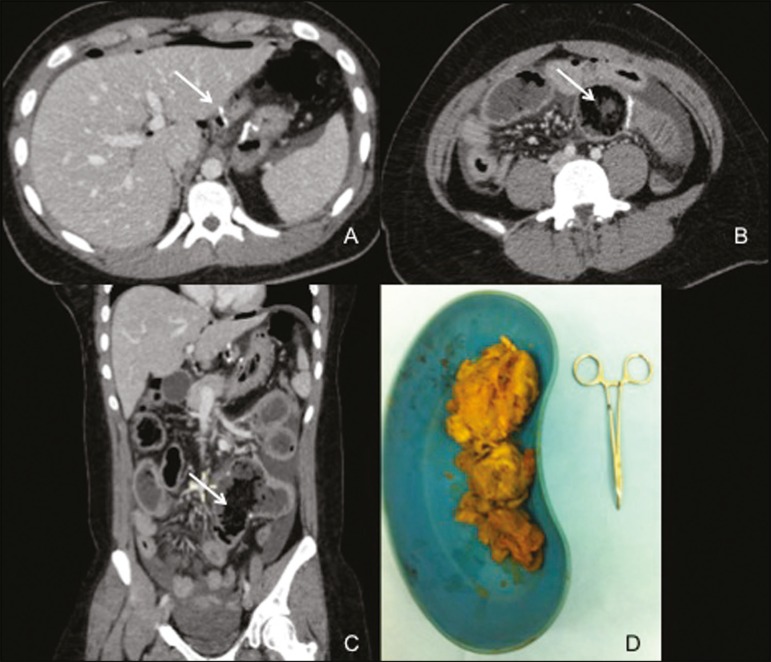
CT of the abdomen and pelvis, with intravenous contrast. A:
